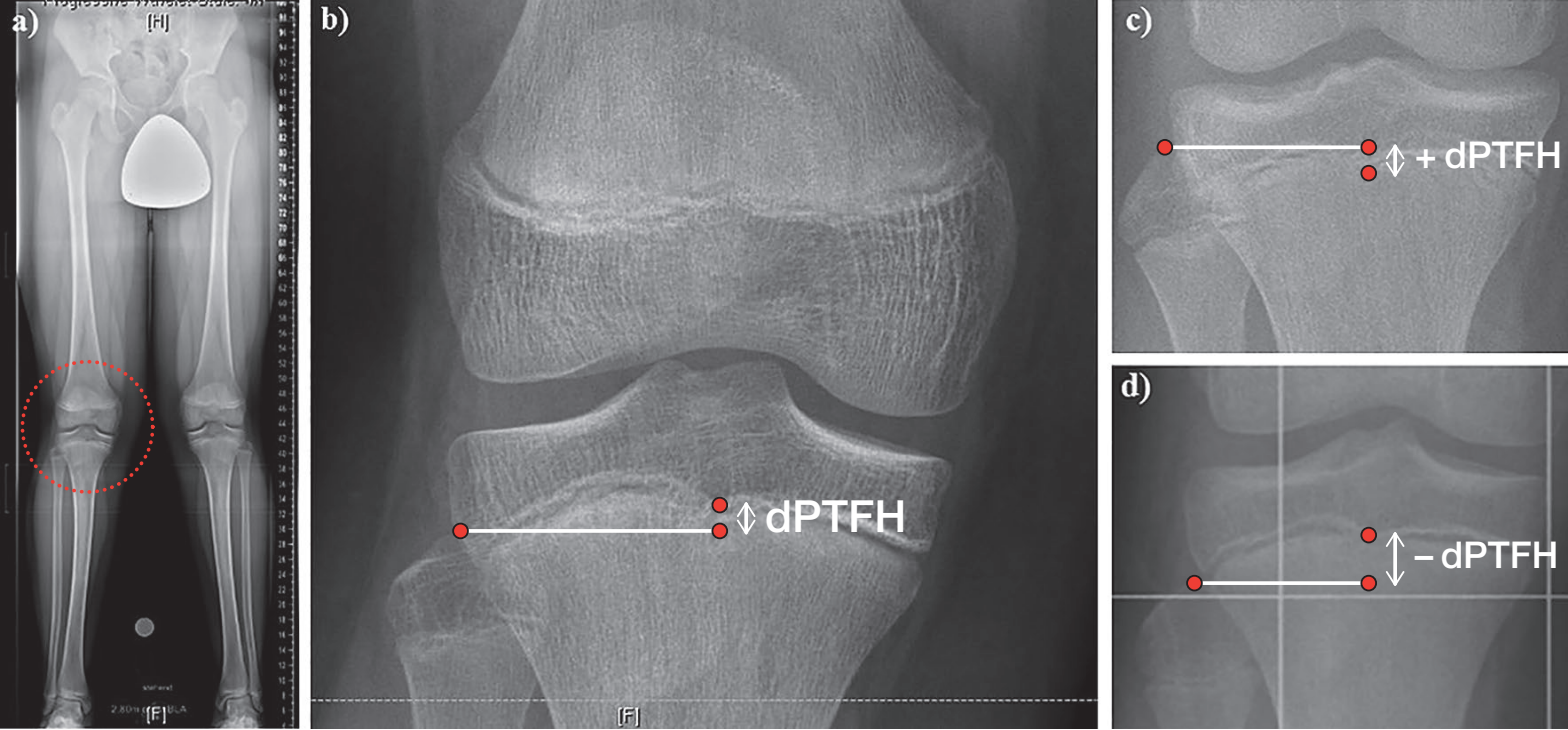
(a) The distance between the center of the proximal tibial growth plate and the tip of the fibular head (dPTFH) is measured in the frontal plane of long standing radiographs of a 13-year-old boy. (b) dPTFH is defined by the distance in millimeters between the center of the tibial growth plate and a line tangential to the tip of the fibular head and horizontal to the imaging plane. (c, d) Negative values indicate that the fibular head is localized more distally than the center of the proximal tibial growth plate and vice versa.
